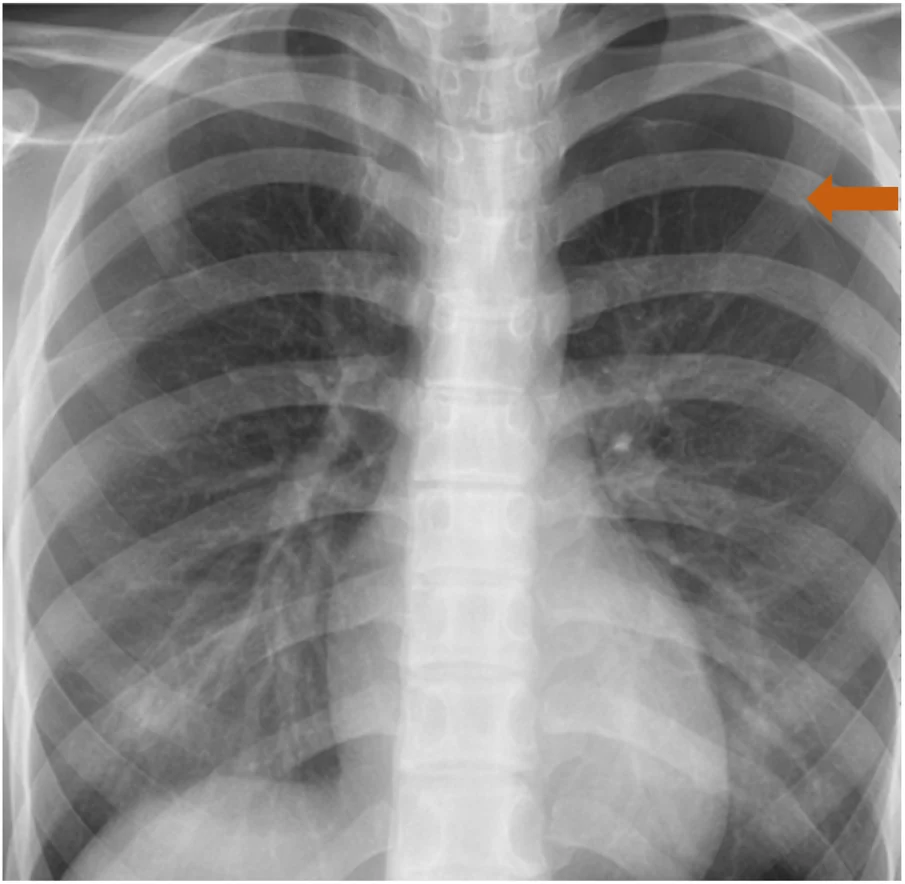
Chest x-ray before the second surgery, confirming left spontaneous pneumothorax.
